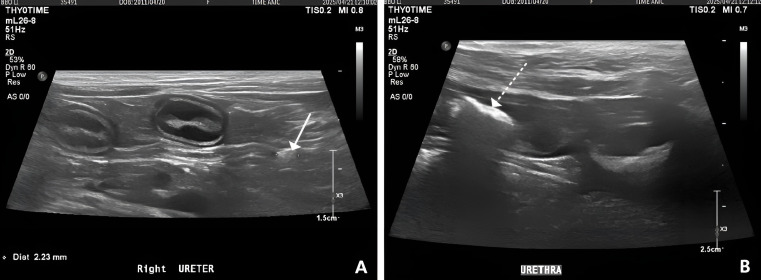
Ultrasonographic images of ureteral and urethral calculi. (A) A urolith (arrow with line) measuring approximately 2.23 mm in diameter was identified within the right ureter. (B) A urolith (arrow with dotted line) was observed within the left ureter.
